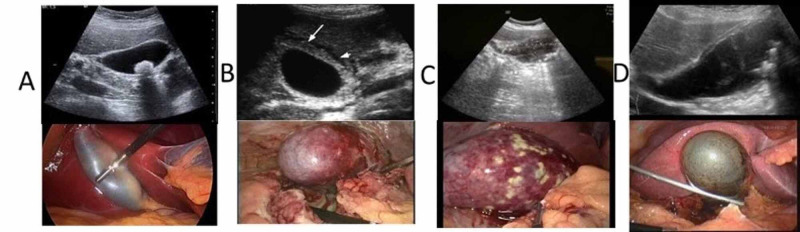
Ultrasonographic findings and the corresponding intra-operative images of (A) normal gallbladder, (B) acute cholecystitis, (C) gallbladder empyema and (D) gangrenous cholecystitis.
